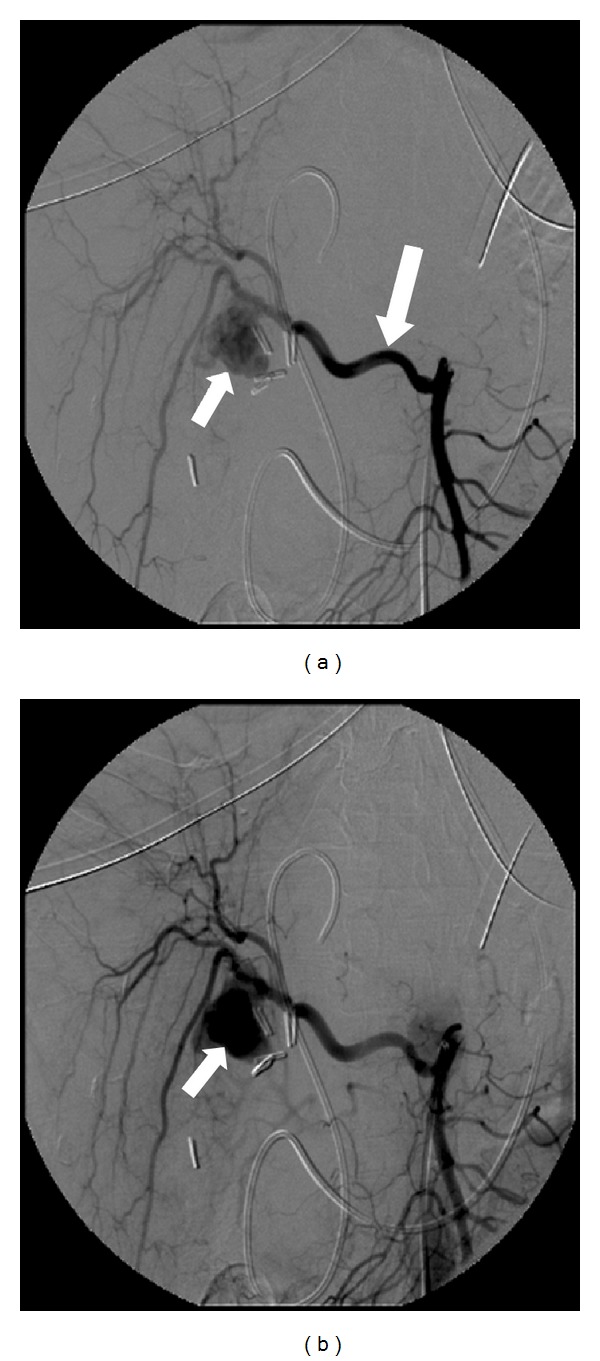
The angiogram shows the sac of a 2 cm pseudoaneurysm, with no radiologic evidence of arterobiliary fistula. A few days before, the CT scan revealed a vascular lesion of 4 mm, successively enlarging to the size reached at the moment of bleeding (smaller arrow). The pseudoaneurysm was located on a replaced right hepatic artery branching off the superior mesenteric artery (greater arrow).
